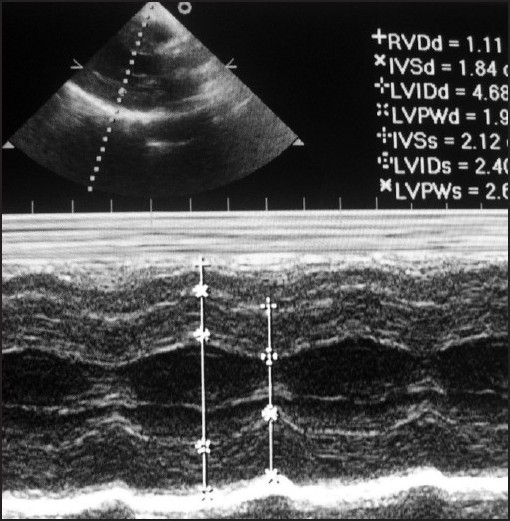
Echocardiogram of patient with severe aortic stenosis
